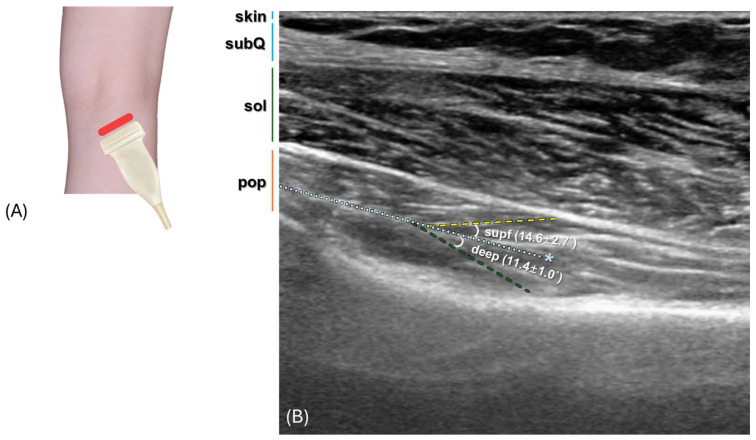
Ultrasound image of the popliteus muscle. (A) Probe positioning for oblique scanning at three standardized locations on the popliteal fossa. (B) Ultrasound image on B site. The popliteus muscle was divided into a superficial (yellow line) and a deep (green line) layer based on the central tendon (asterisk). subQ, subcutaneous tissue, sol, soleus muscle, pop, popliteus muscle.
